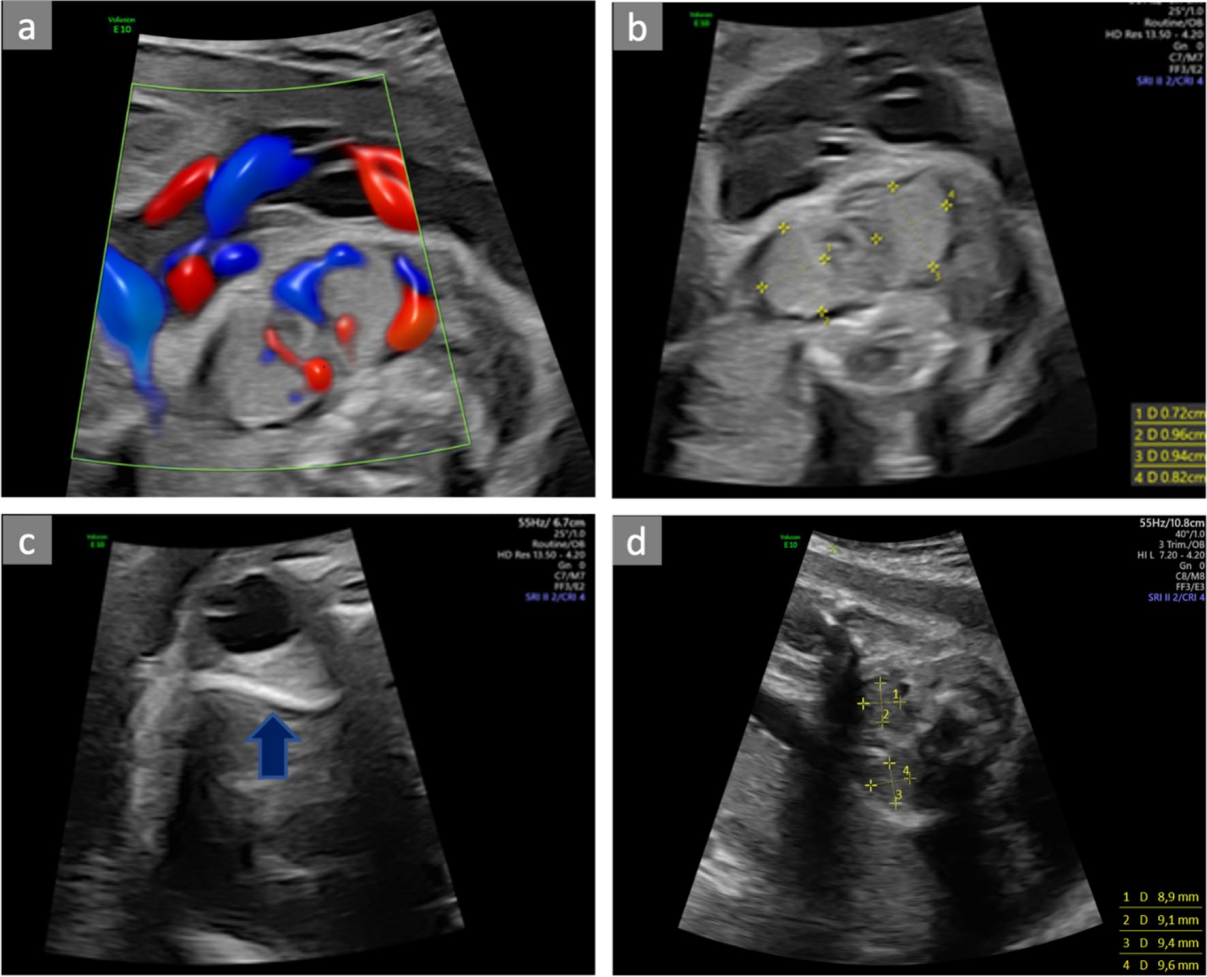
a, b Transverse plane of the thyroid gland of the fetus. a Color Doppler imaging demonstrates the increased blood flow to the enlarged glands despite high PRF. b Biometry shows the enlagerment of thyroid glands. c Image of the exophthalmus showing the enlarged area behind the left bulbus (blue arrowhead). d Image of the thyroid gland in transverse plane at 26 + 4 weeks. Usually, further growth of the thyroid gland would be expected [4]. However, as a result of successful treatment with PTU, the gland´s size remains constant (95th percentile). By 29 + 4 weeks, the thyroid diameter was within normal range again
